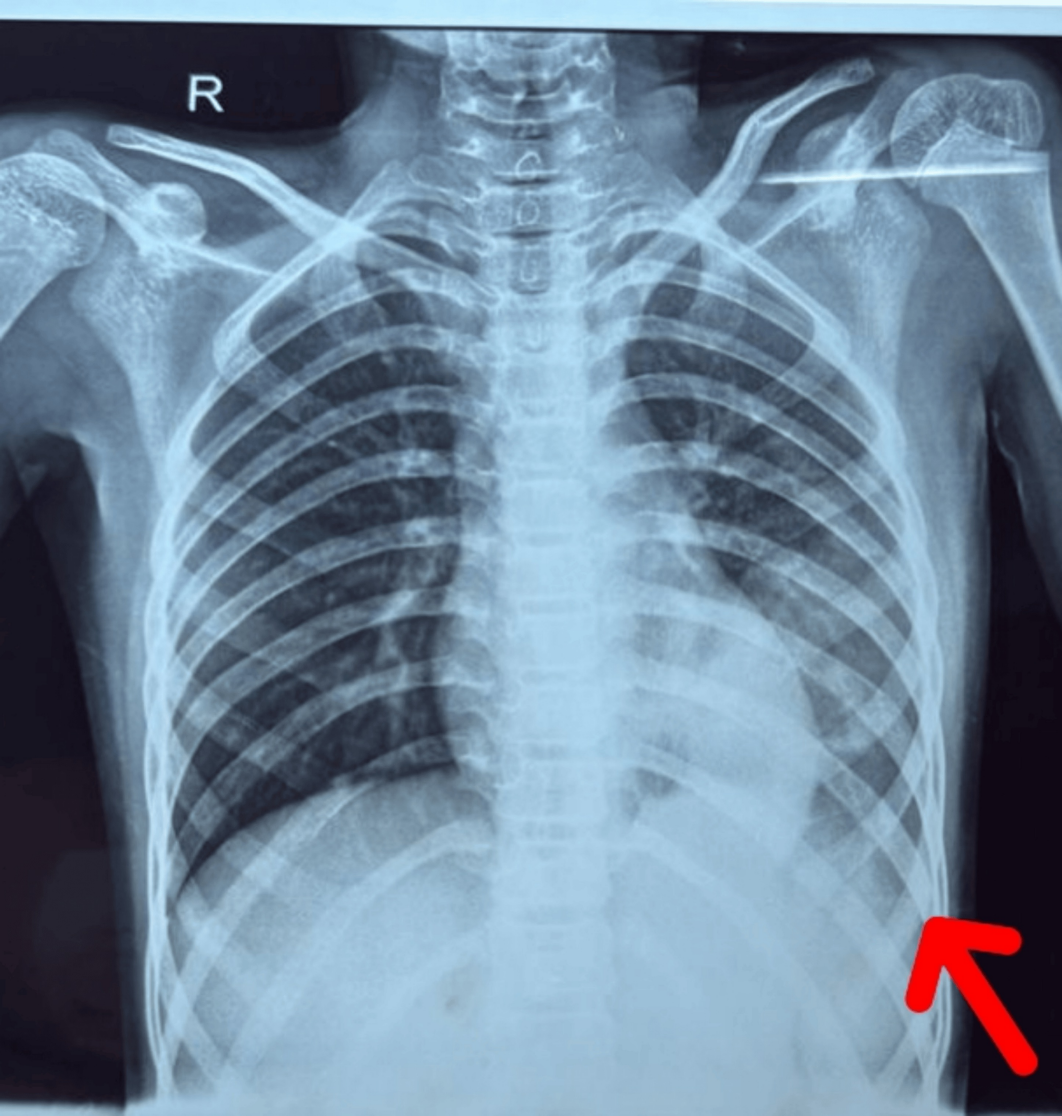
X-ray chest showing left lower lobe consolidation.
